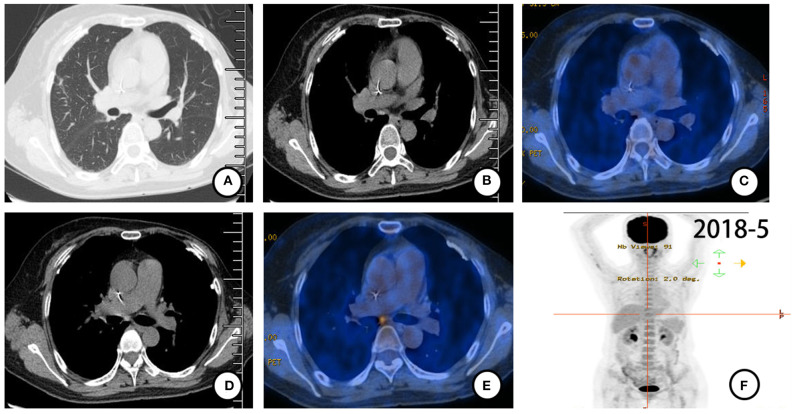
PET-CT (May 2018) scan after 6 cycles of combination treatment with trastuzumab, vinorelbine, and capecitabine. (A–F) All metastases shrank with almost no FDG uptake.
